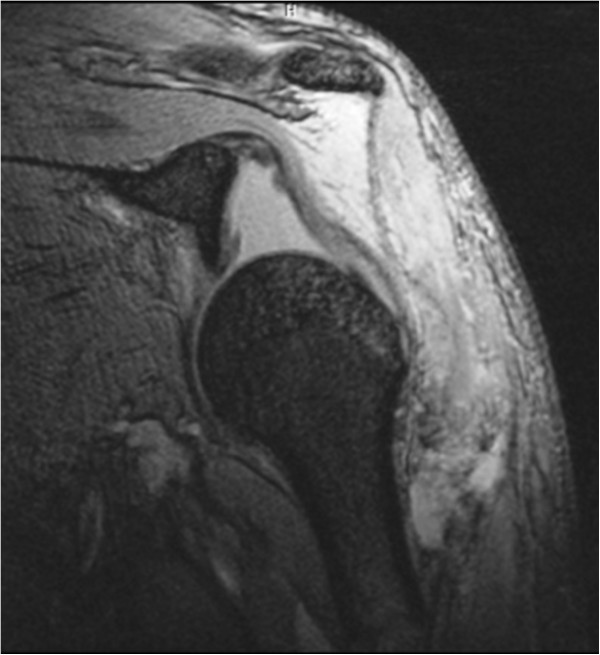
Coronal section of magnetic resonance imaging showing shoulder dislocation and intact rotoator cuff.
